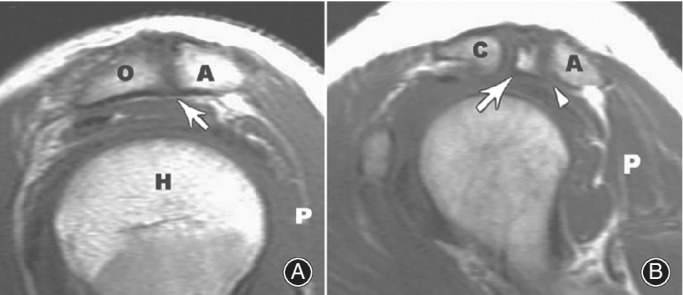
(A) Os acromiale without step‐off deformity. T1‐weighted sagittal MRI of the shoulder shows an os acromiale without step‐off deformity (arrow) relative to the inferior cortex of the acromion. A, acromion; H, humeral head; O, os acromiale; P, posterior. (B) Os acromiale with step‐off deformity. T1‐weighted sagittal MRI of the shoulder shows malalignment (white arrowhead) between the inferior cortex of the os acromiale (arrow) and the acromion. A, acromion; C, clavicle; P, posterior^24^.
